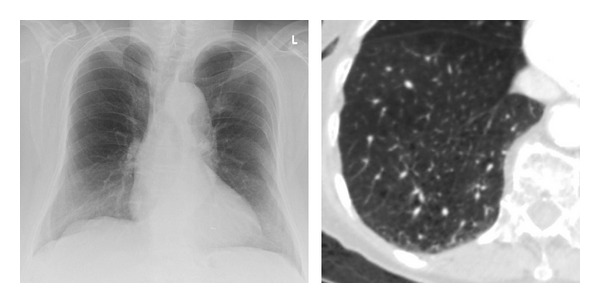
Senile emphysema in an 88-year-old patient. Chest X-ray (on the left) and centrilobular emphysematous changes on computed tomography (on the right). Imaging was ordered because of suspected mesenterial ischemia.
